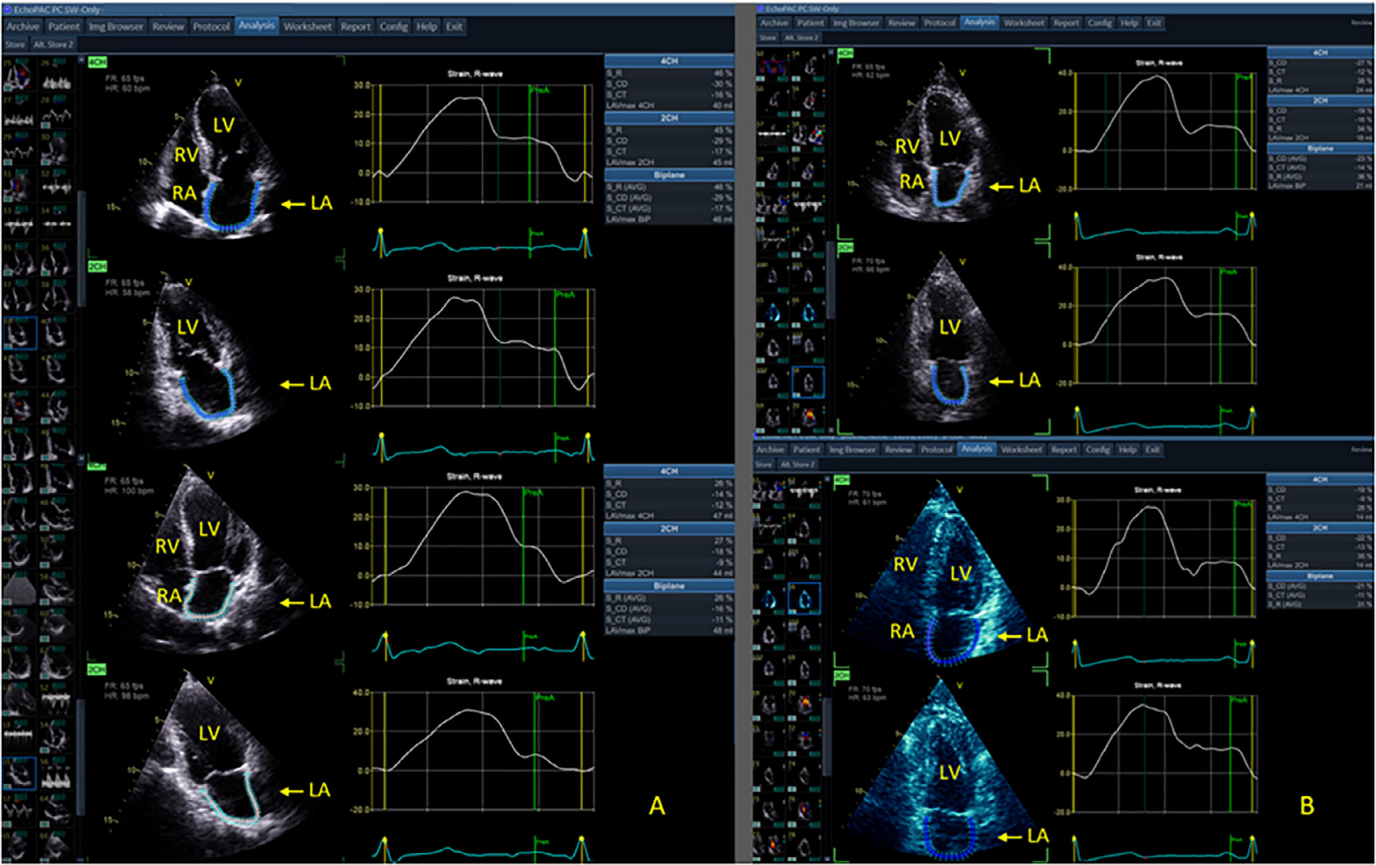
General Electric EchoPAC left atrial strain before and after exercise. (A) Patient with an ischemic stress echocardiogram (Ischemic SE); (B) Patient with a non‐ischemic stress echocardiogram but abnormal diastolic stress test (abnormal DST). DST, diastolic stress test; SE, stress echocardiogram.
